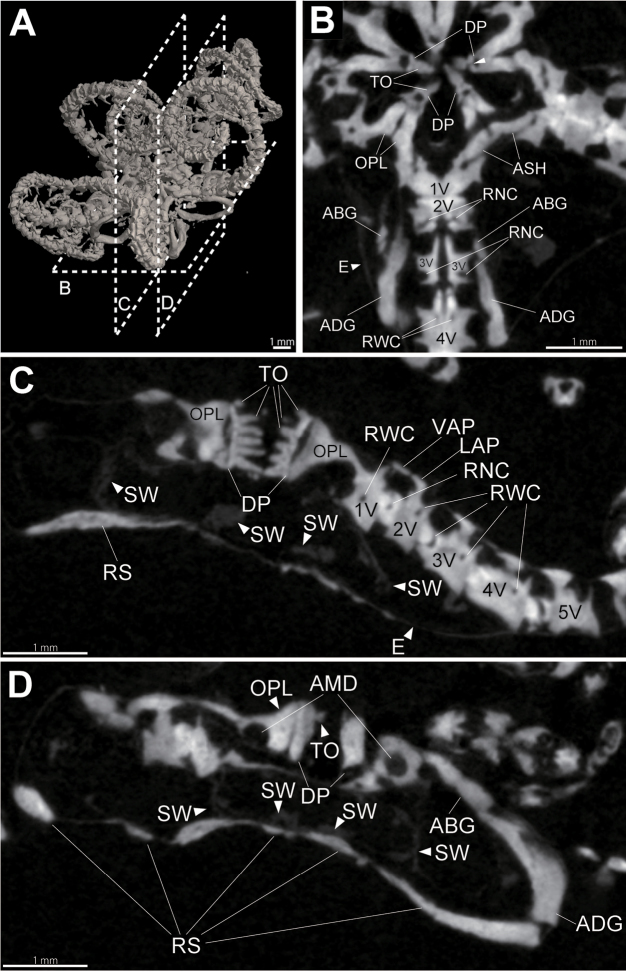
Micro CT surface rendered (A) and 2D section (B–D) images of the entire body of *Asteronyx
